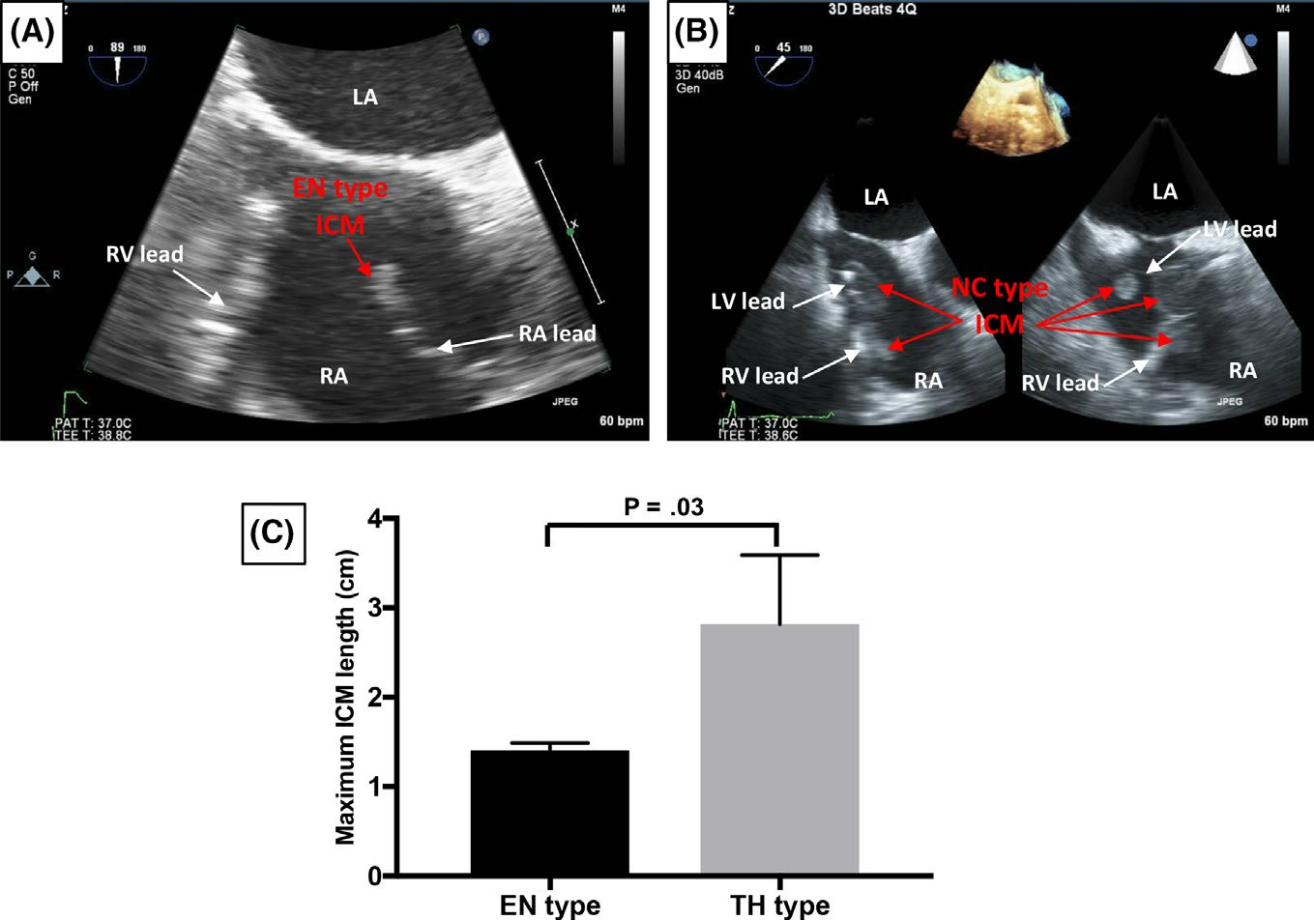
Echocardiographic study of EN type intracardiac mass (ICM) and NC type ICM. A, The EN type ICM is shown. The typical EN type ICM appears as a solitary caulescent round shape. B, The NC type ICM is shown. The typical NC type ICM appears as a multilocular lobular shape. There are multiple ICMs in this view. C, Comparison of ICM maximum length between EN type and NC type ICMs. The NC type is significantly longer (2.8 ± 0.7 cm) than the EN type (1.4 ± 0.1 cm, *P* = .03). Abbreviations: RA, right atrium; LA, left atrium; RV, right ventricle; LV, left ventricle
